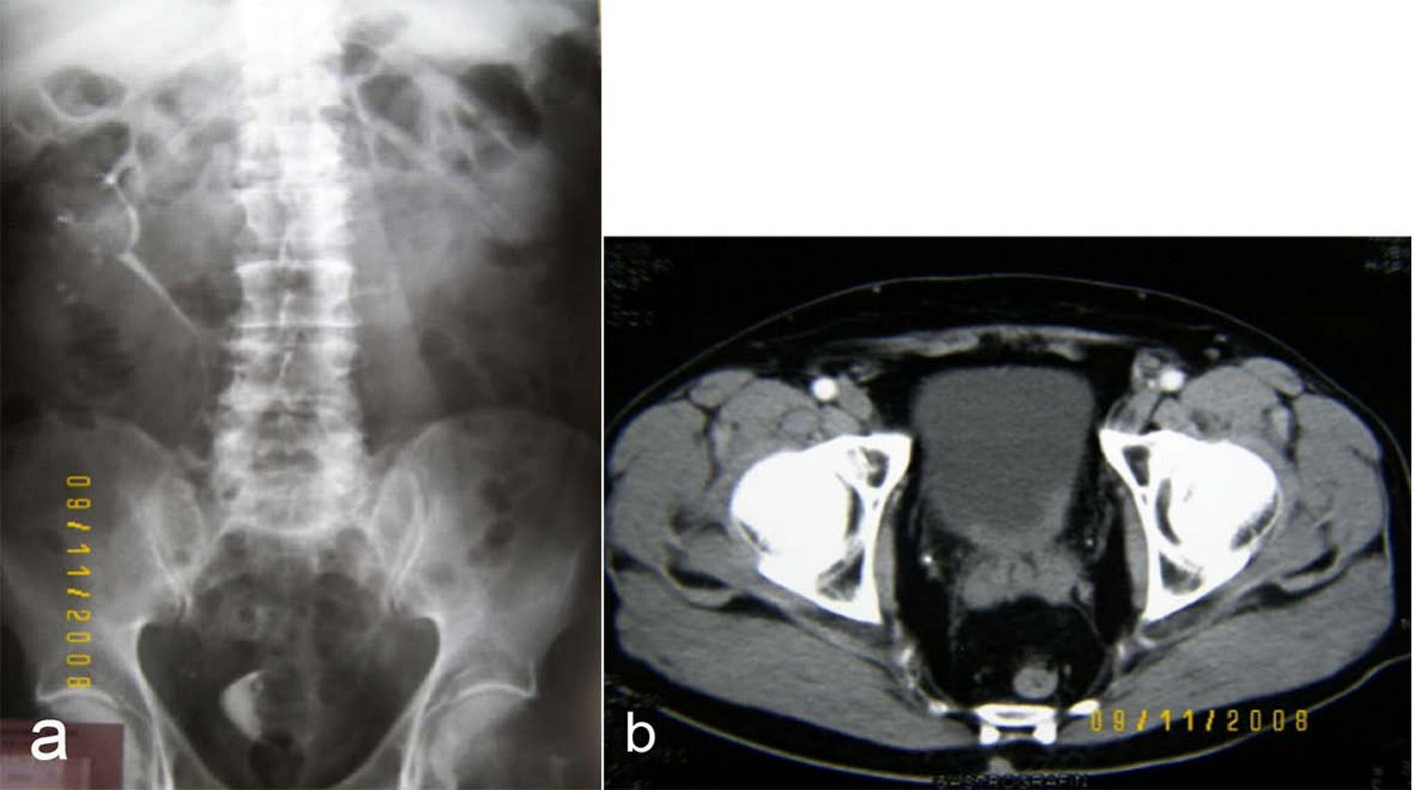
a, Intravenous urogram showing a radiolucent filling defect in the bladder and a non functioning left kidney and b, computed tomography of the pelvis.
